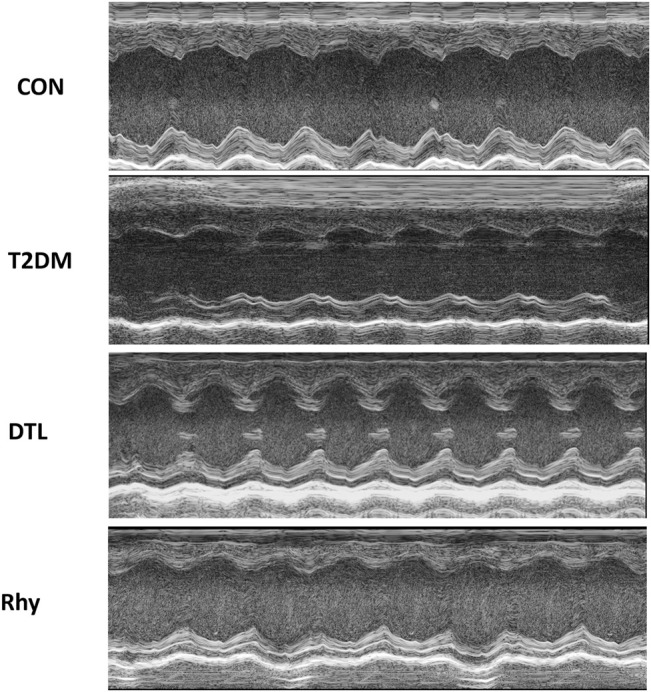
The effects of rhynchophylline on the echocardiographic parameters. Representative M-mode images of left ventricular diameters and wall thicknesses.
